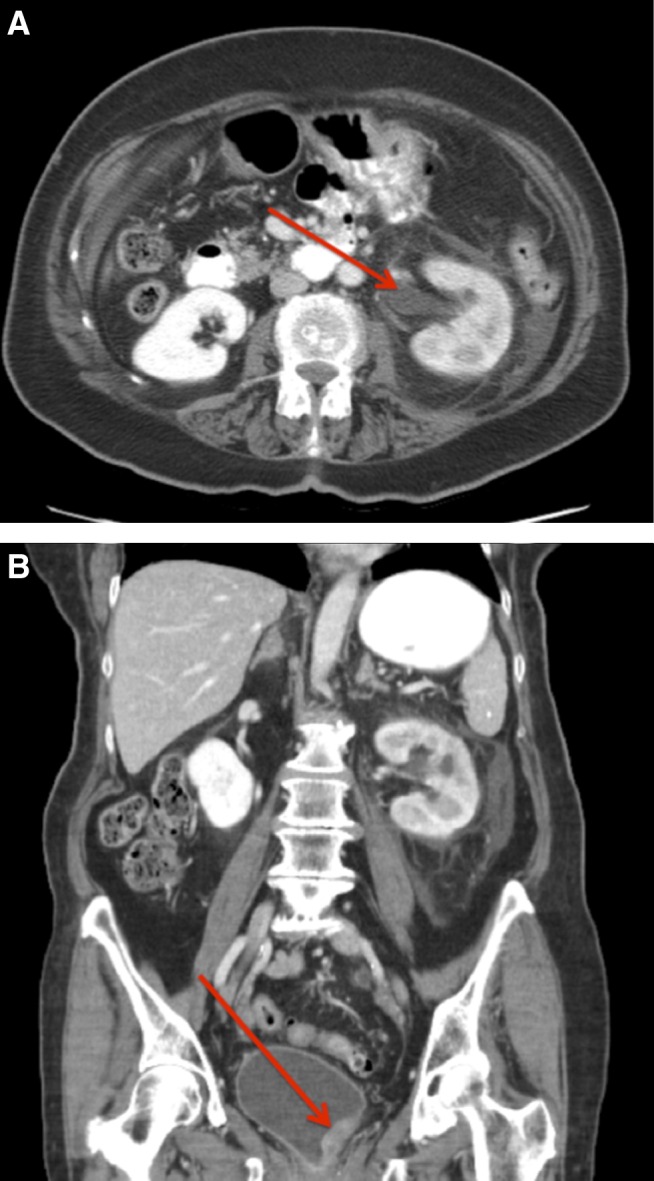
Abdominal and pelvic CT scan revealing left-sided hydroureteronephrosis and delayed nephrogram, suggesting obstruction (A) and bladder mass (B) appearing to obstruct the left ureteral orifice.
